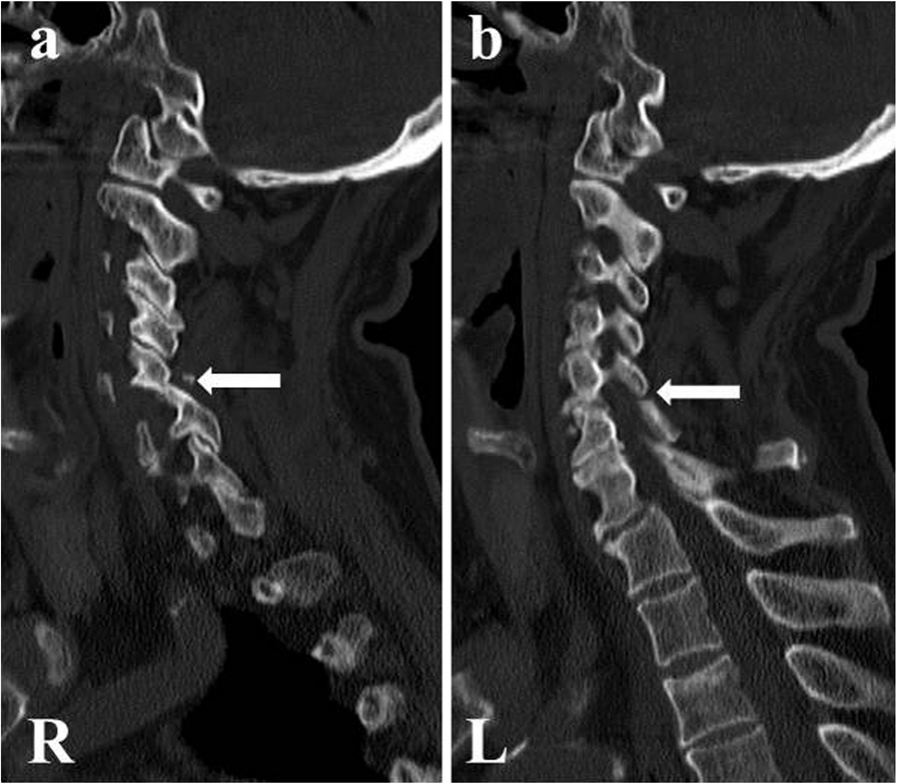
Sagittal computed tomography performed eight weeks after the traffic accident showing dislocation of both facet joints at C5 to C6. (a) R: right side (arrow). (b) L: left side (arrow).
